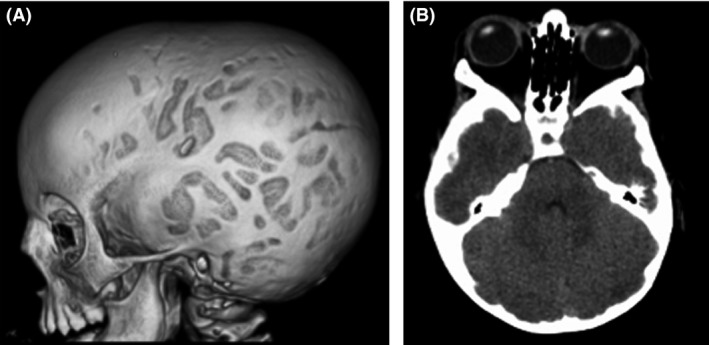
Radiologic data of the index patient (III:4) (A) craniosynostosis with digital impressions on cranial three‐dimensional CT scan. (B) CT scan at 20 months showing bilateral proptosis of grade 2 secondary to the craniosynostosis.
